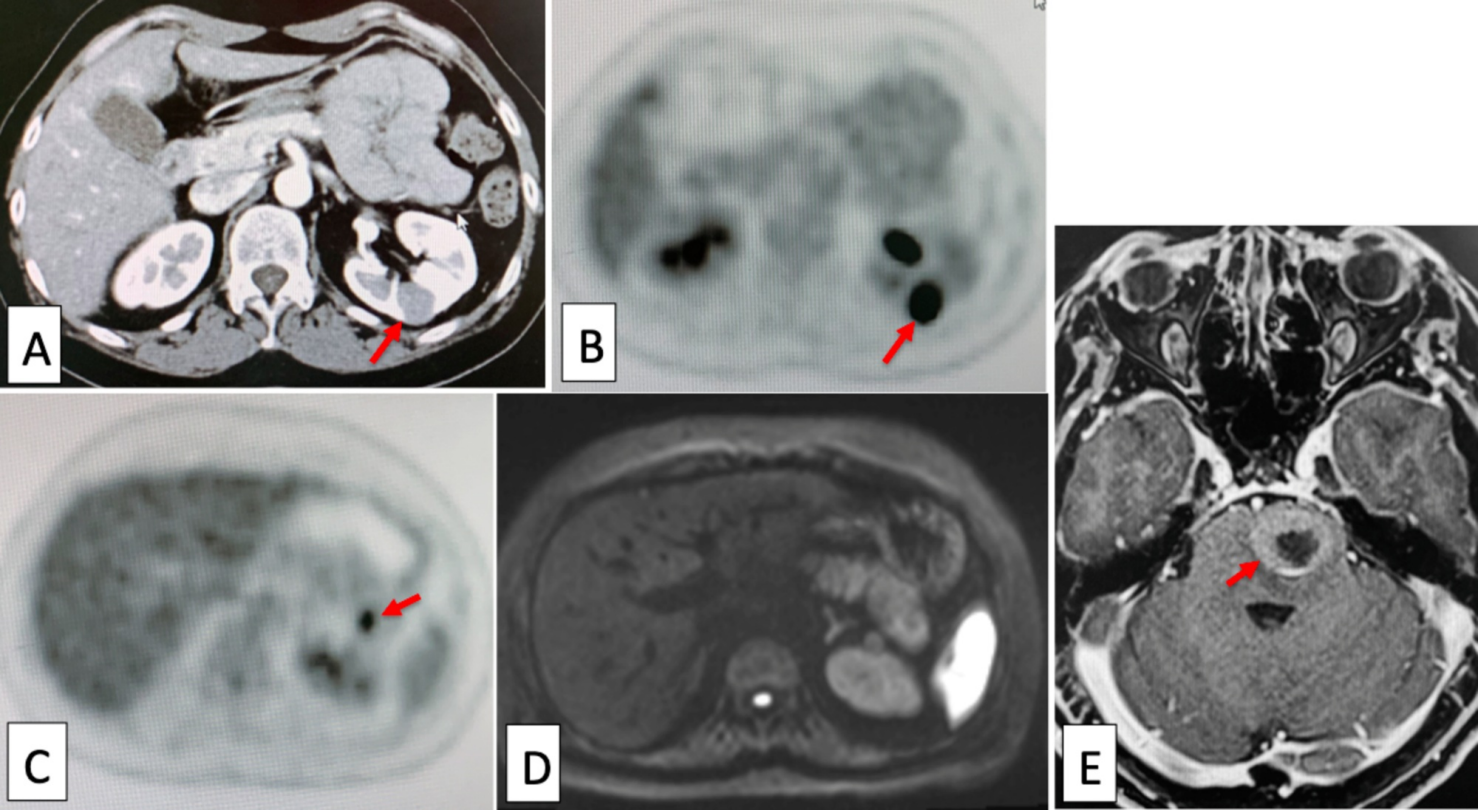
Left kidney, pancreas, and brain metastases Computed tomography (CT) showed a mass in the left kidney (A, arrow), which showed high-uptake of FDG (2-[18F]fluoro-2-deoxy-D-glucose) in positron emission tomography (FDG-PET) (B, arrow). FDG-PET also revealed an FDG high-uptake lesion in the pancreas (C, arrow). The pancreatic lesion was obscure on CT and magnetic resonance imaging (MRI) (D). Brain MRI showed a metastasis with ringed gadolinium contrast enhancement in the pons (E, arrow).
